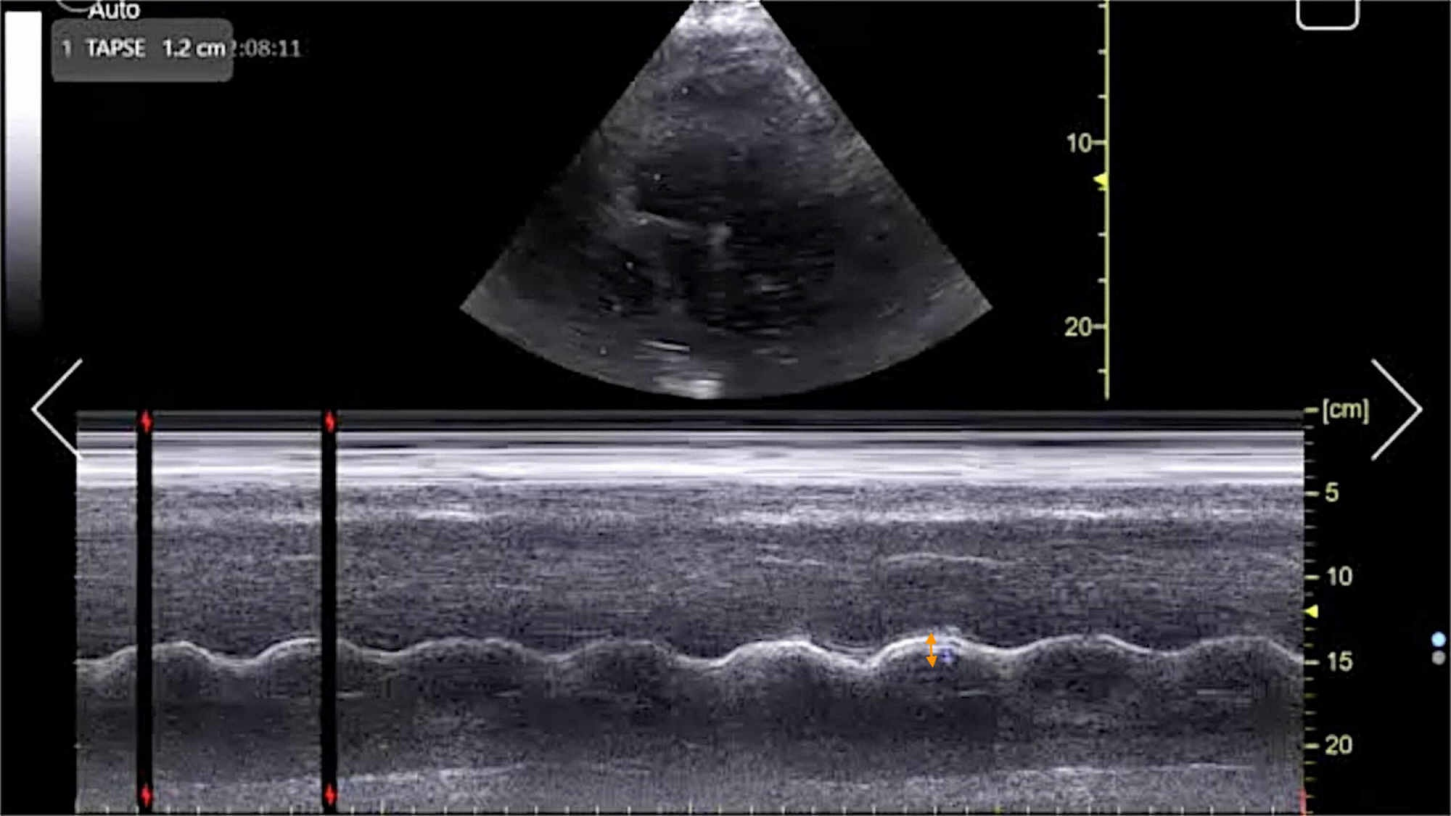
Point-of-care echocardiography pre-thrombolysis. Apical four-chamber view with M-mode tracing demonstrating TAPSE (orange arrow). TAPSE, tricuspid annular plane systolic excursion.
